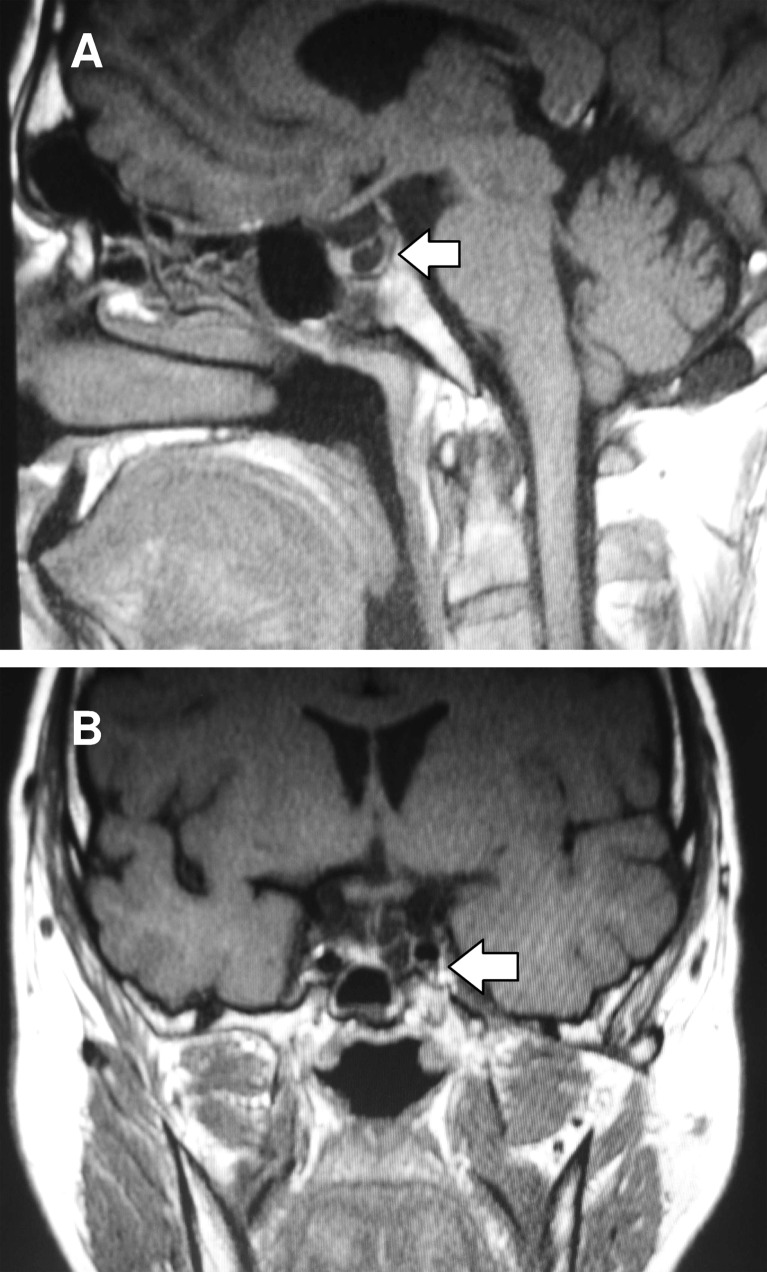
a Midline sagittal and b coronal magnetic resonance imaging (MRI) sections showing hypoplasia of the pituitary anterior lobe and presence of an anterior lobe cyst in patient 1
